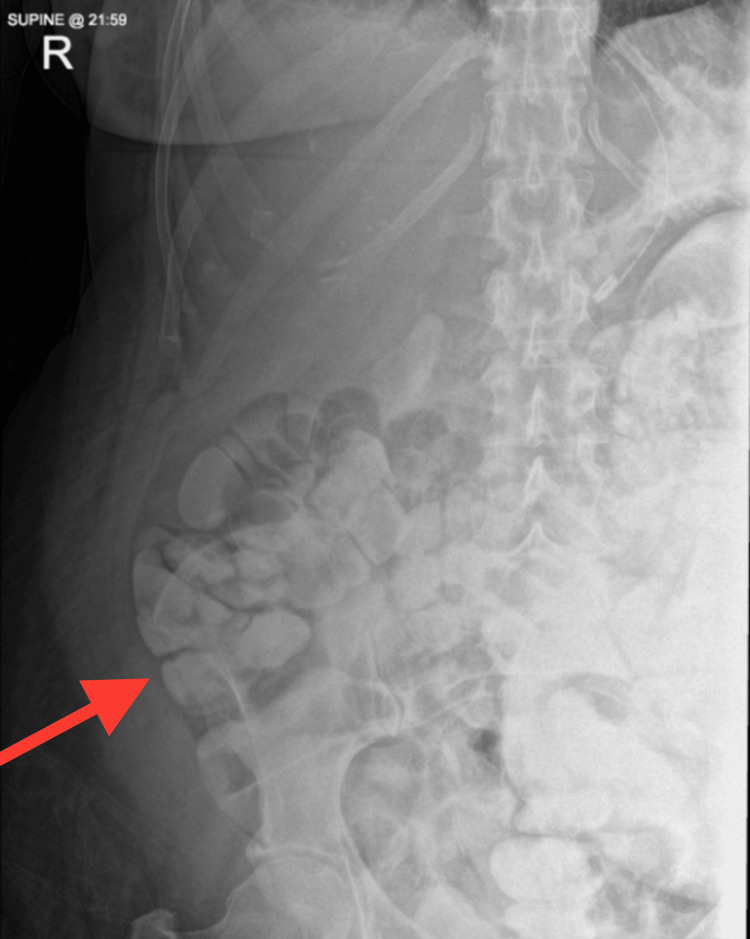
Clinical resolution was apparent on abdominal X-ray by day 8 after surgery Abdominal radiograph on postoperative day 8 showing resolution of the small bowel obstruction. The previously distended loops have decompressed, indicating restoration of bowel continuity and function after corticosteroid therapy
